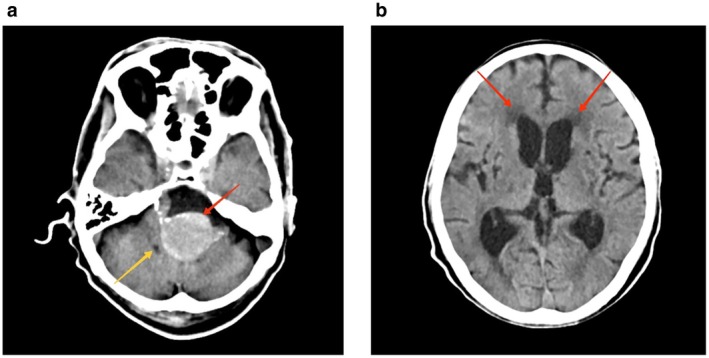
(a) Non‐contrast CT head axial section at the level of 4th ventricle showing hyperdense lesion (red arrow) is noted anterior to the brainstem with a marked mass effect in the form of compression of 4th ventricle (yellow arrow). (b) Non‐contrast CT head axial section at level of lateral ventricles showing dilated bilateral lateral ventricles with periventricular seepage (red arrow) suggestive of hydrocephalus.
